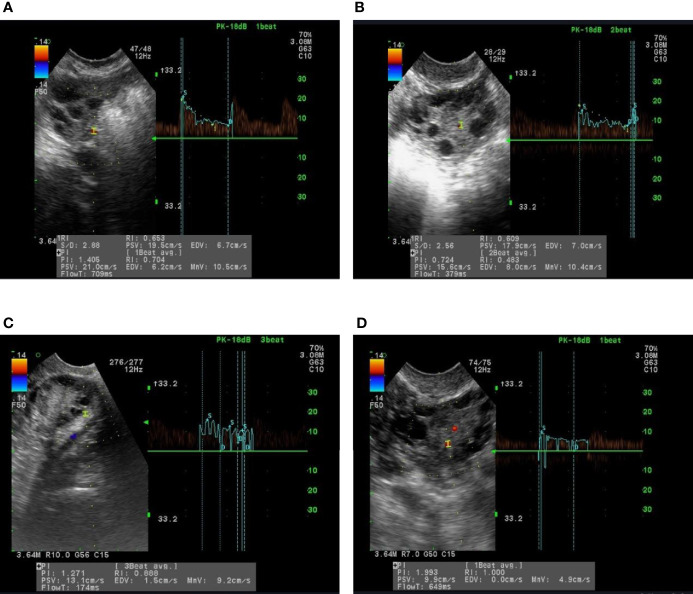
Images of ultrasound recordings of ovarian blood flow among PCOS patients after Diane-35 treatment. Images (A–D) are of representative patients in groups (A–D), as described in the methods section.
